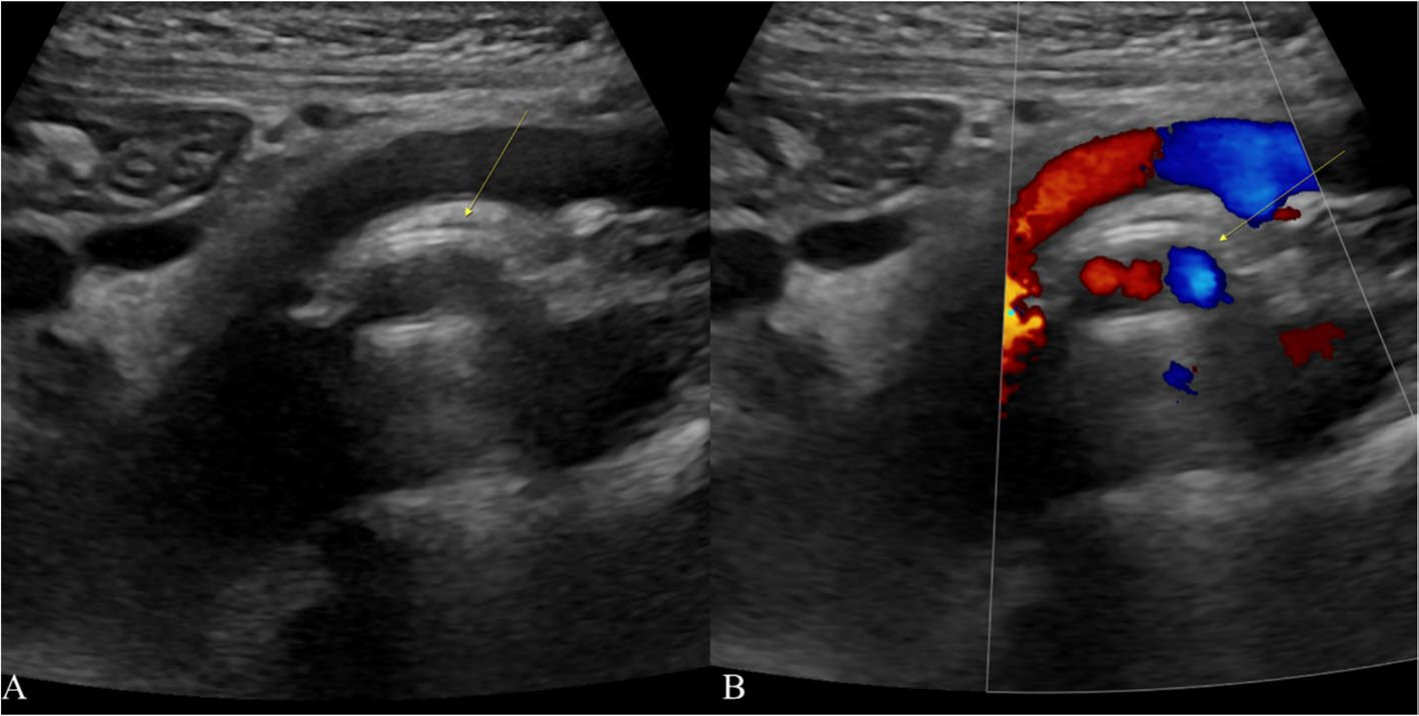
The 3D-printed PEEK extravascular stent in the same patient (arrow): A B-mode ultrasound in the sagittal plane, B Doppler ultrasound in the sagittal plane
